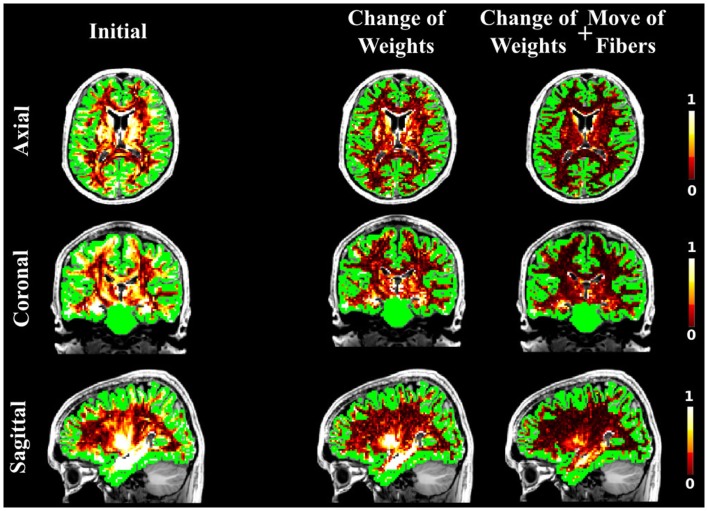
The NMSE maps between the acquired *in vivo* dMRI image and the signal generated with the initial set derived from the STREAMLINE tracking. The maps are overlayed onto the T1-weighted and GM segmentation in green. The first column shows the NMSE for the starting set, the second column shows the results when running our method by only changing weights, and the last when all our proposals are used in the optimization.
